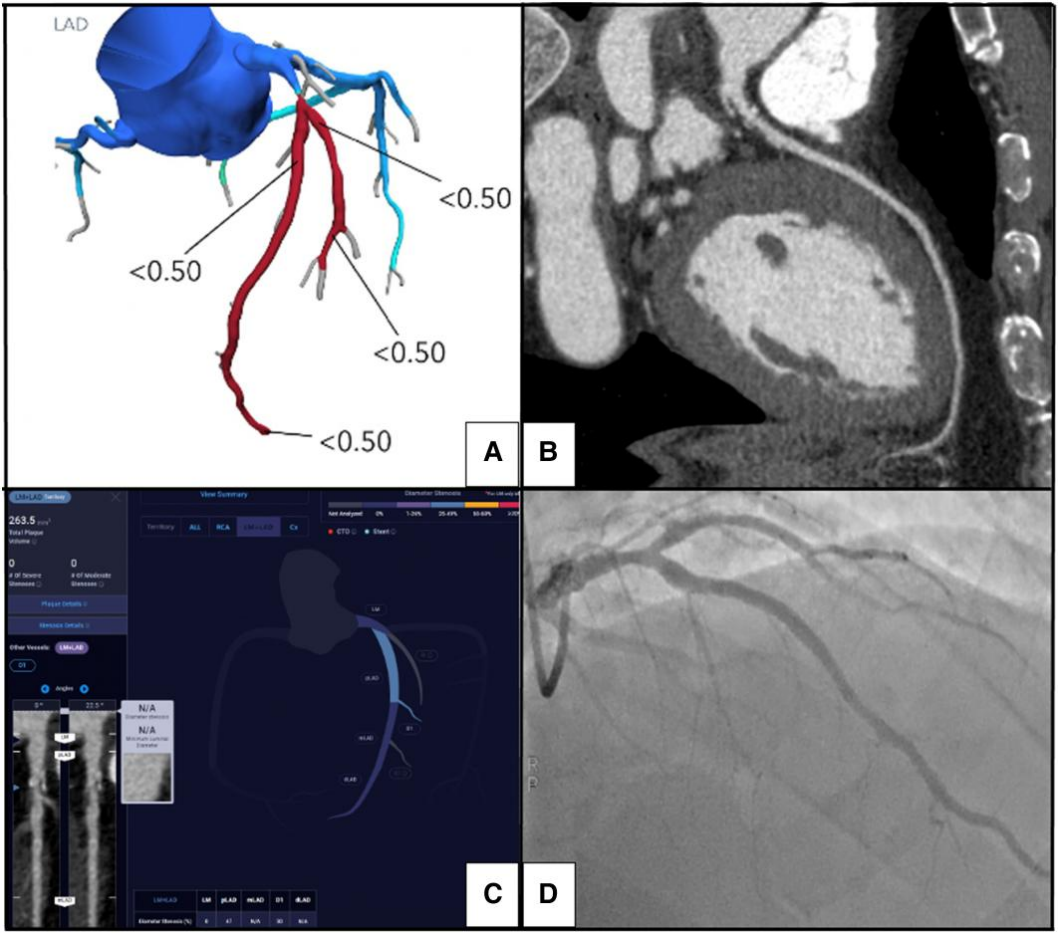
Representative example of all metrics assessed including CT-FFR, expert physician visual interpretation of CAD-RADS, AI-QCT_ISCHEMIA_, and ICA. (*A*) CT-FFR analysis of proximal LAD with a value < 0.50; (*B*) expert physician interpretation of CCTA showing moderate stenosis proximal LAD with MD read of CAD-RADS 3; (*C*) AI-QCT analysis of proximal LAD reading mild stenosis without significant ischaemia; (*D*) ICA of the proximal LAD with mild disease and physiologically non-significant ICA-FFR value of 0.95. CT-FFR, coronary computed tomography angiography using fractional flow reserve; AI-QCT, artificial intelligence coronary computed tomography; ICA, invasive coronary angiography.
